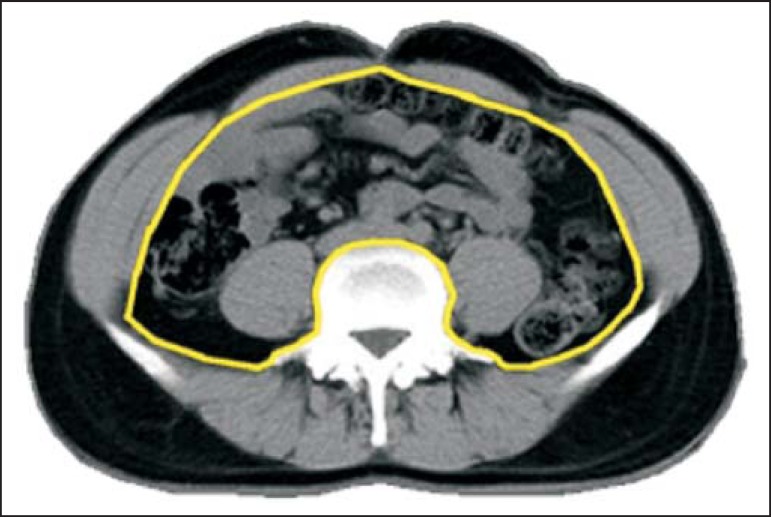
Axial CT scan after manual segmentation of the peritoneal anatomical
